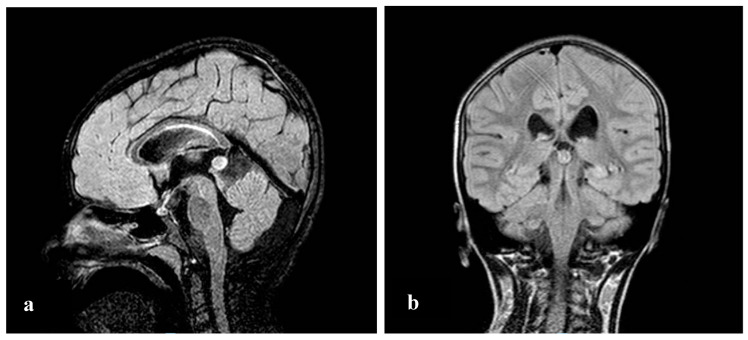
Poly-lobed cystic pineal gland in sagittal (a) and coronal (b) T2 FLAIR sections.
